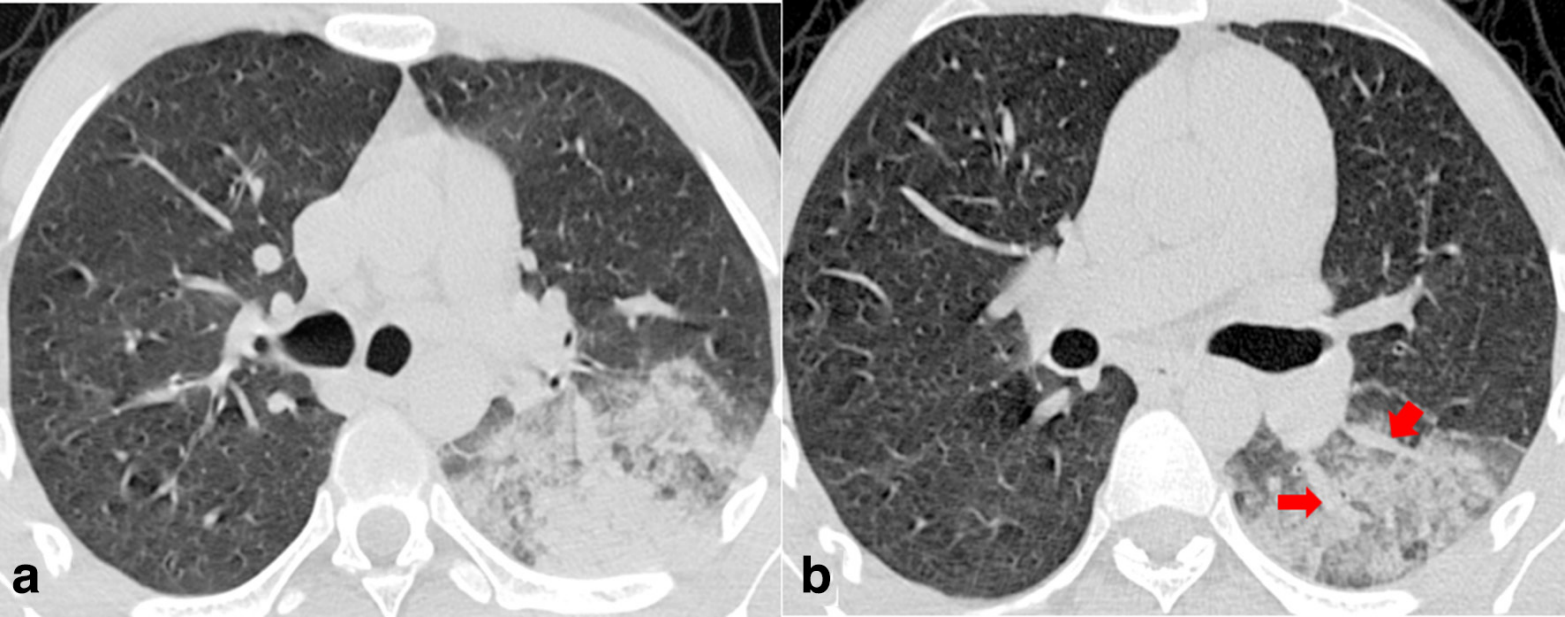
Non-contrast chest CT axial image (a) in a 24-year-old COVID-19 positive male patient showing coalescent consolidative opacity in the superior segment of the left lower lobe. Vessel dilatation sign is seen in (b) denoted by arrow. The patient was clinically unstable and required ventilation in the ICU. COVID-19, Corona virus disease 2019; ICU, intensive care unit.
